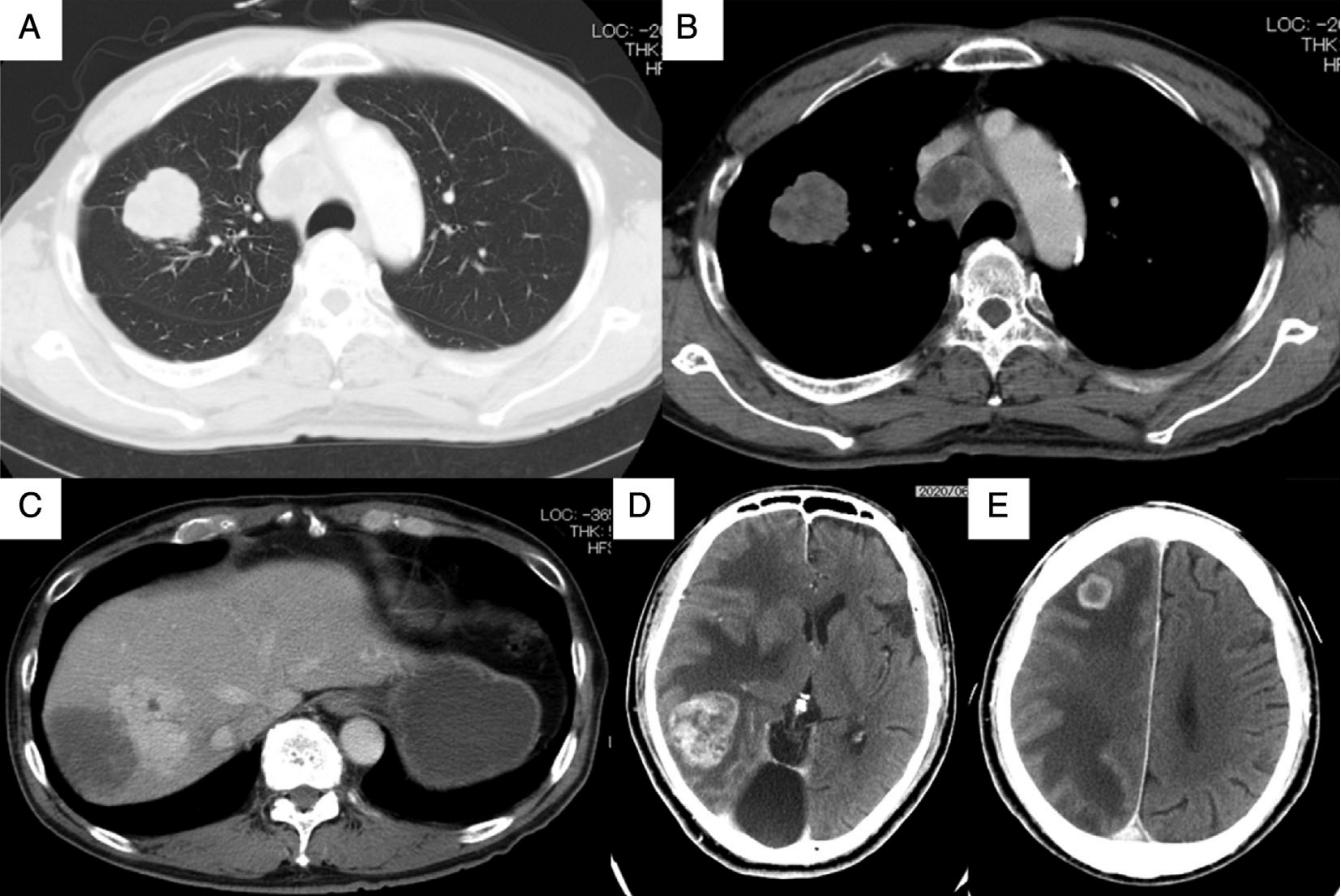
Systemic contrast‐enhanced computed tomography (CT) images before alectinib treatment. (A,B) Chest CT revealed a 38 mm mass in the right upper lobe and multiple mediastinal lymph node metastases. (C) Abdominal CT revealed liver metastases. (D,E) Head CT revealed multiple brain metastases in the right occipital and right parietal lobes
